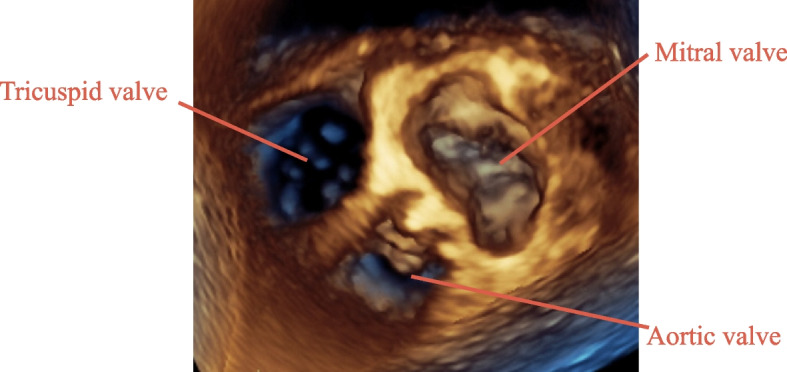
The 3-dimensional echocardiographic image of the tricuspid valve enface view from right atrium. The tricuspid valve is seen to the left, the mitral valve on the right, and the aortic valve below the two valves
